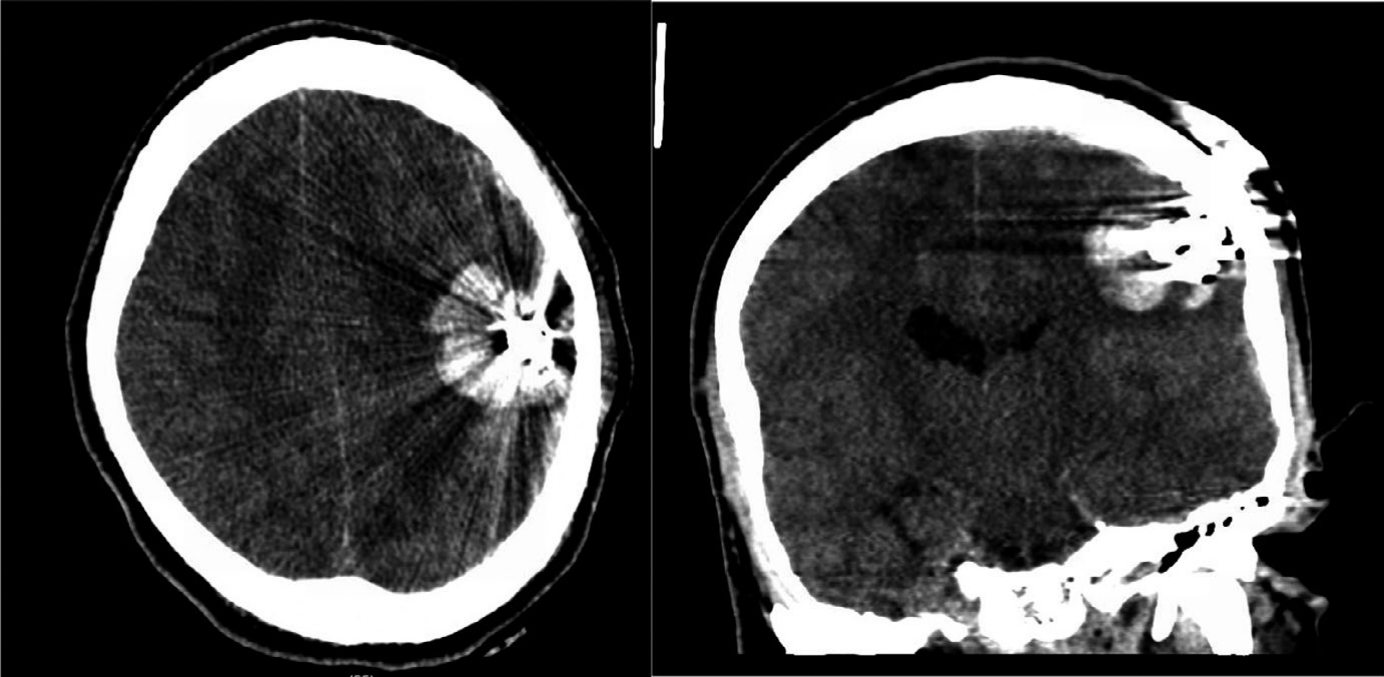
Admission head CT, Axial head computed tomography (left) and coronal head CT (right) demonstrate the bolt penetrating through the left parietal skull into the underlying brain with associated intraparenchymal hemorrhage
